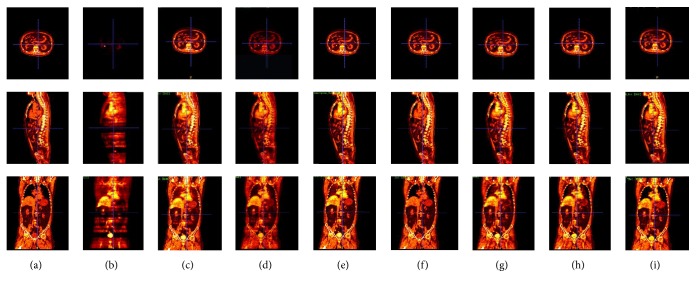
The 3D reconstructions with pseudo-color of the fusion result sequences. The first row represents transverse plane. The second row represents the sagittal plane. The last row represents the coronal plane. (a) and (b) are the 3D reconstructions of the source CT and PET image sequences, respectively. (c)–(i) are the reconstructions of the fusion result sequences that are produced by (c) DWT, (d) NSCT, (e) NSCT_PCNN_1, (f) NSCT_SF_PCNN, (g) NSCT_PCNN_2, (h) NSCT_PCNN_3, and (i) the proposed framework, respectively.
